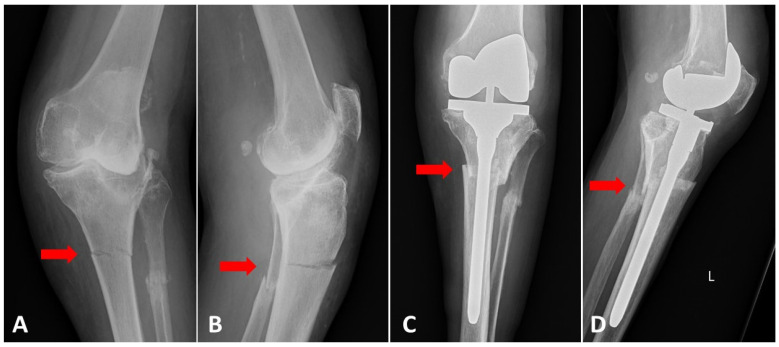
(A) AP and (B) lateral radiograph of the patient with the fracture of 1/3 proximal of the tibial shaft with coexisting severe osteoarthritis and valgus knee. Postoperative (C) AP and (D) lateral knee radiographs. The fracture was fixated intramedullary with the use of a well-fitted long-stemmed TKA. Postoperative radiograph indicates that the use of 4 mm tibial offset on the lateral site direction could improve the alignment of bone fragments.
